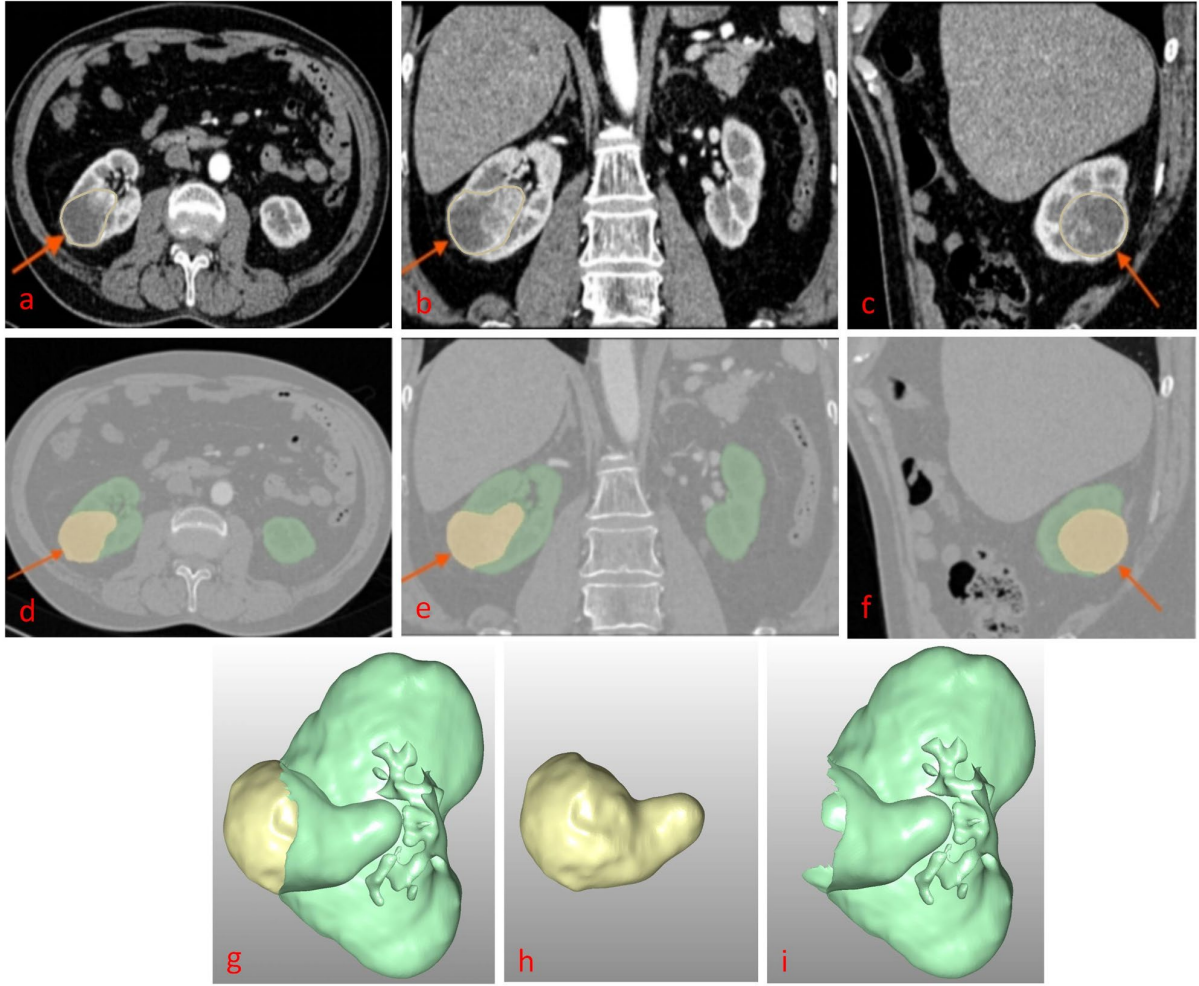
A 70-year-old male with incidentally detected right renal clear cell carcinoma (pathological stage T1b, grade 3). Panels a, b, and c display original axial, coronal, and sagittal CT arterial-phase images, respectively (red arrows: tumor). Panels d, e, and f show corresponding axial, coronal, and sagittal views of the AI-annotated kidney and tumor contours (red arrows: tumor). Panels g, h, and i present 3D reconstructed segmentation labels for the kidney, tumor, and their combined overlay, respectively
